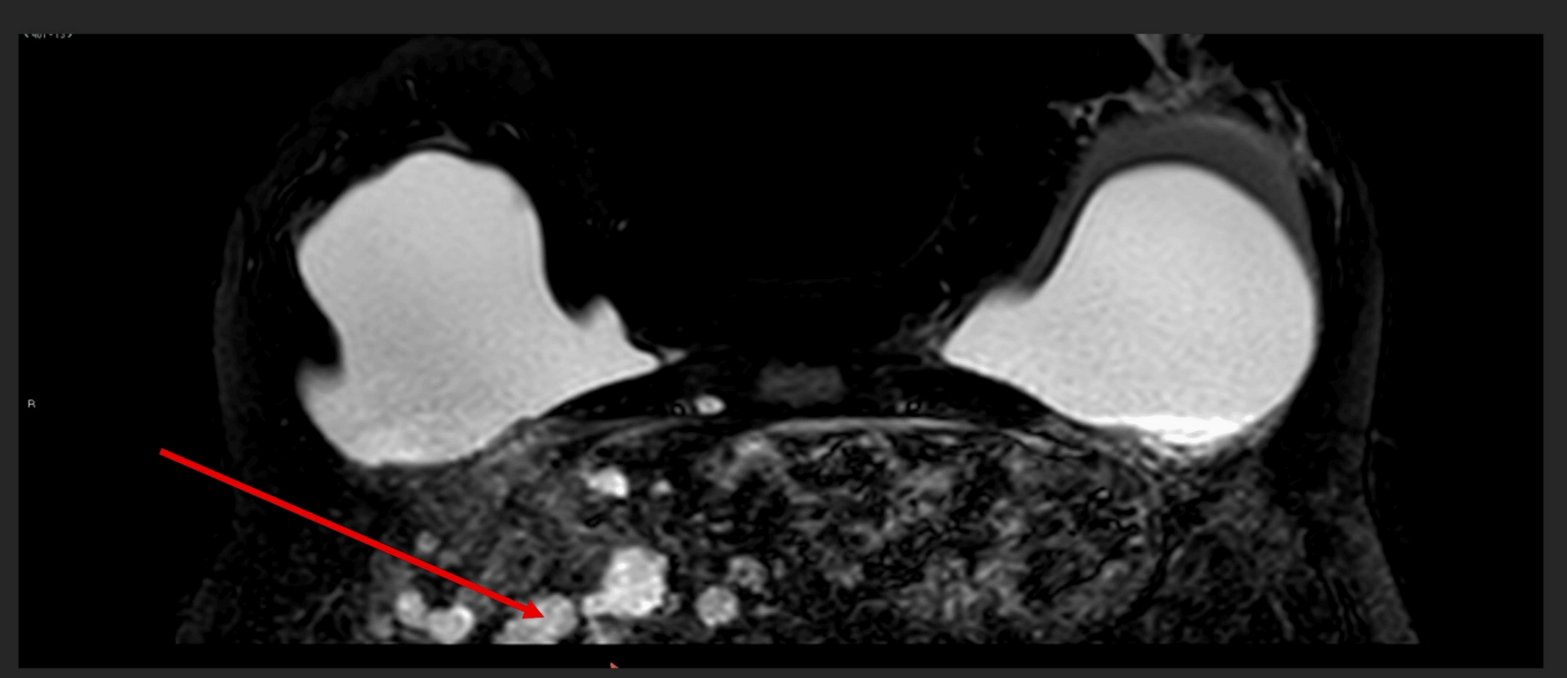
MRI scan of breasts detected silicone containing granulomata (arrow) within the liver incidentally. MRI: magnetic resonance imaging
